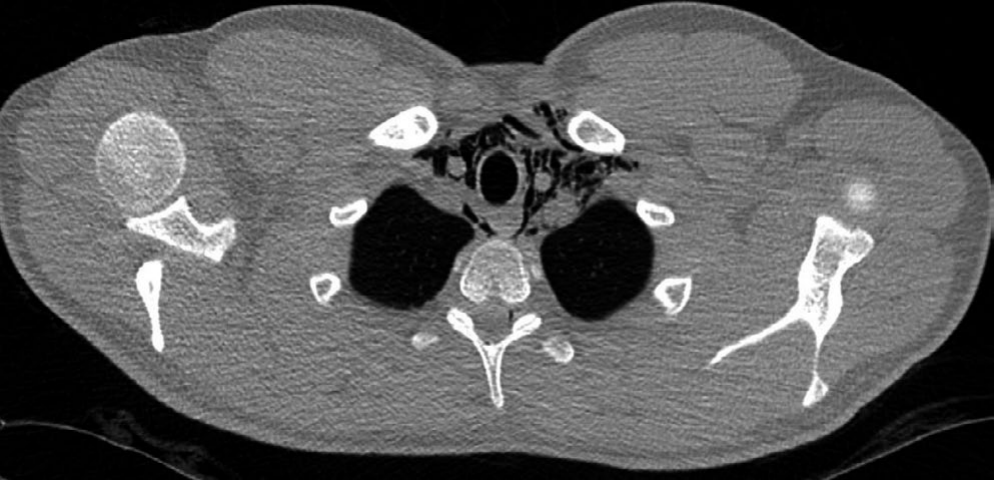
CT scan demonstrating pneumomediastinum
